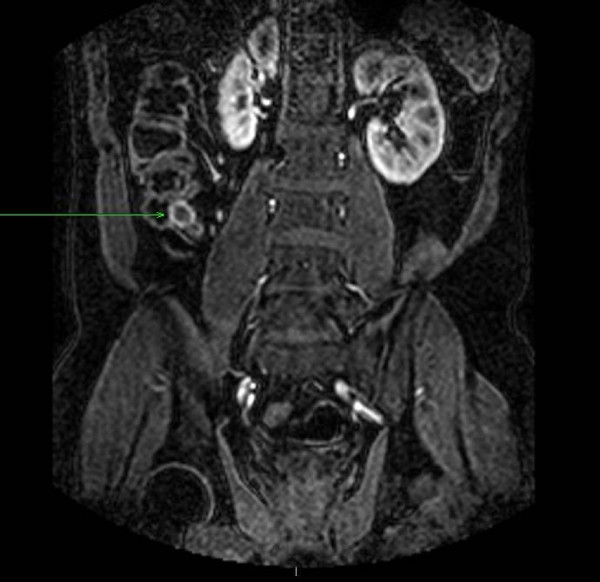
MRI enteroclysis. Green arrow shows a 22 mm peripherally enhancing soft tissue lesion in the right iliac fossa.
